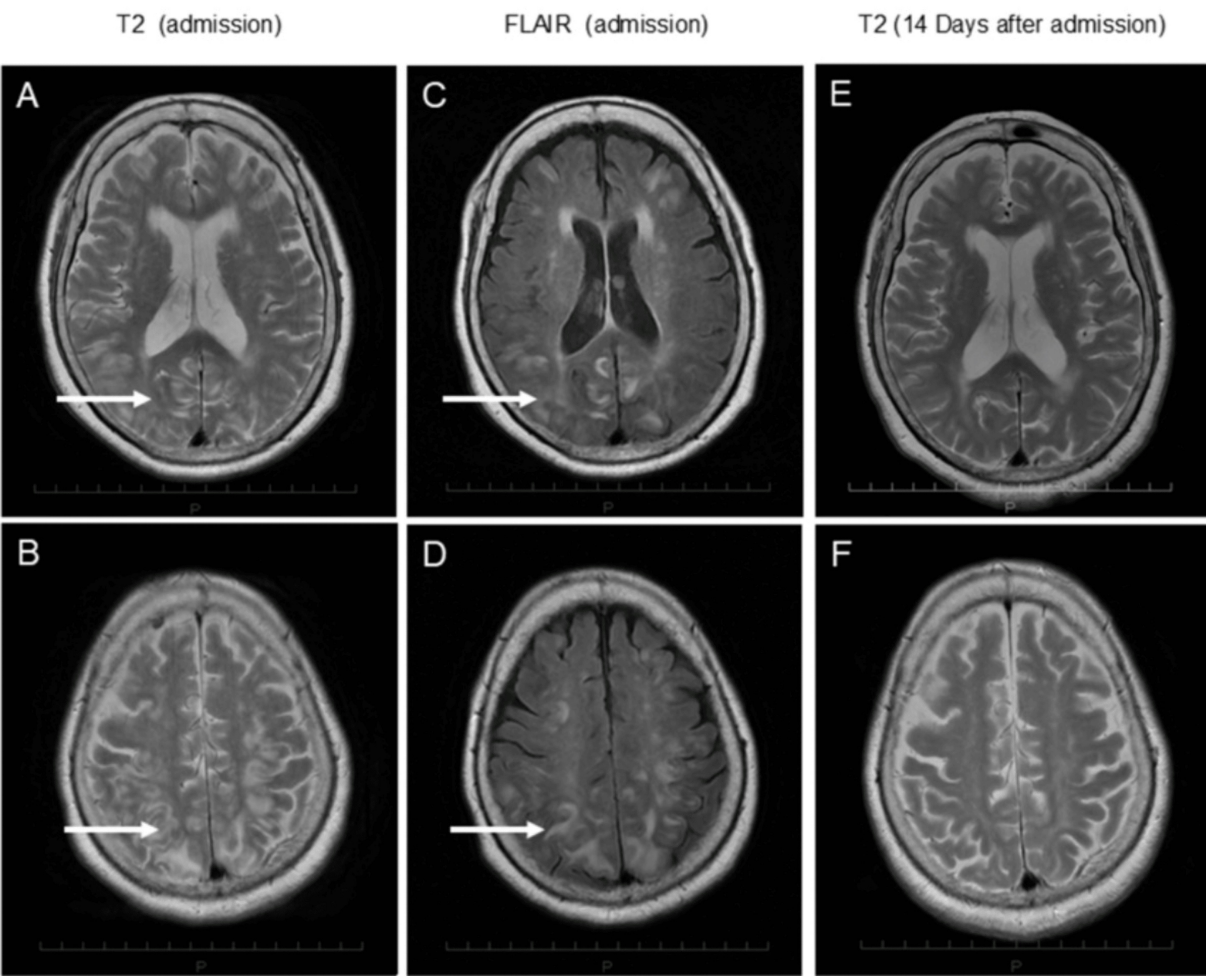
Brain MRI showing posterior vasogenic edema T2-weighted images (A,B) and FLAIR images (C,D) showing bilateral parieto-occipital subcortical hyperintensities consistent with vasogenic edema (white arrows). Follow-up MRI on day 14 demonstrating marked resolution of the lesions (E,F) MRI, magnetic resonance imaging; FLAIR, fluid-attenuated inversion recovery
